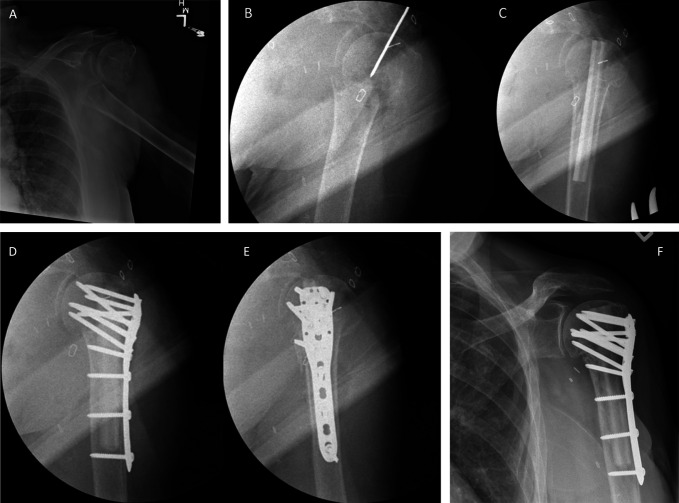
Radiograph demonstrating the case example of a 72-year-old woman who sustained a fall with a resultant displaced proximal humerus fracture of the left upper extremity (A). At presentation, the patient also had a pulseless hand. After an emergent revascularization of the axillary artery, stabilization of the fracture was completed using our technique (B through E). At one year, the patient demonstrated no findings of osseous collapse and maintained stable internal fixation (F).
